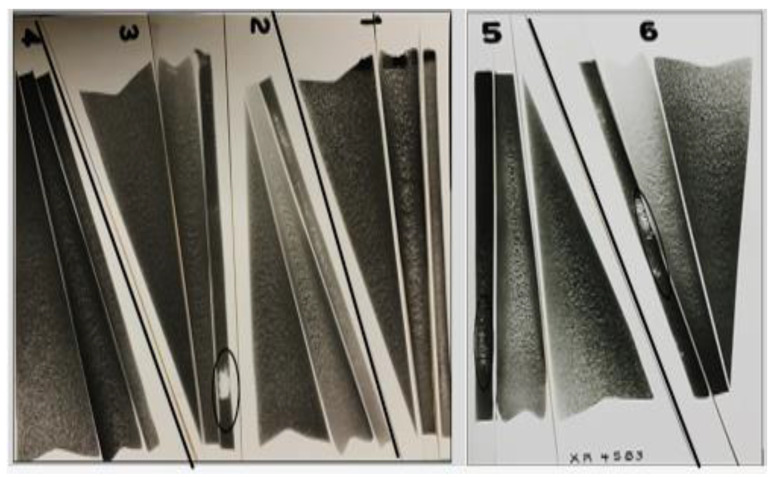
X-ray radiographs taken from several castings at the three opening angles shown in Figure 1e. The numbers 1 to 6 simply represent a batch of castings taken from a specific melt in each case.
